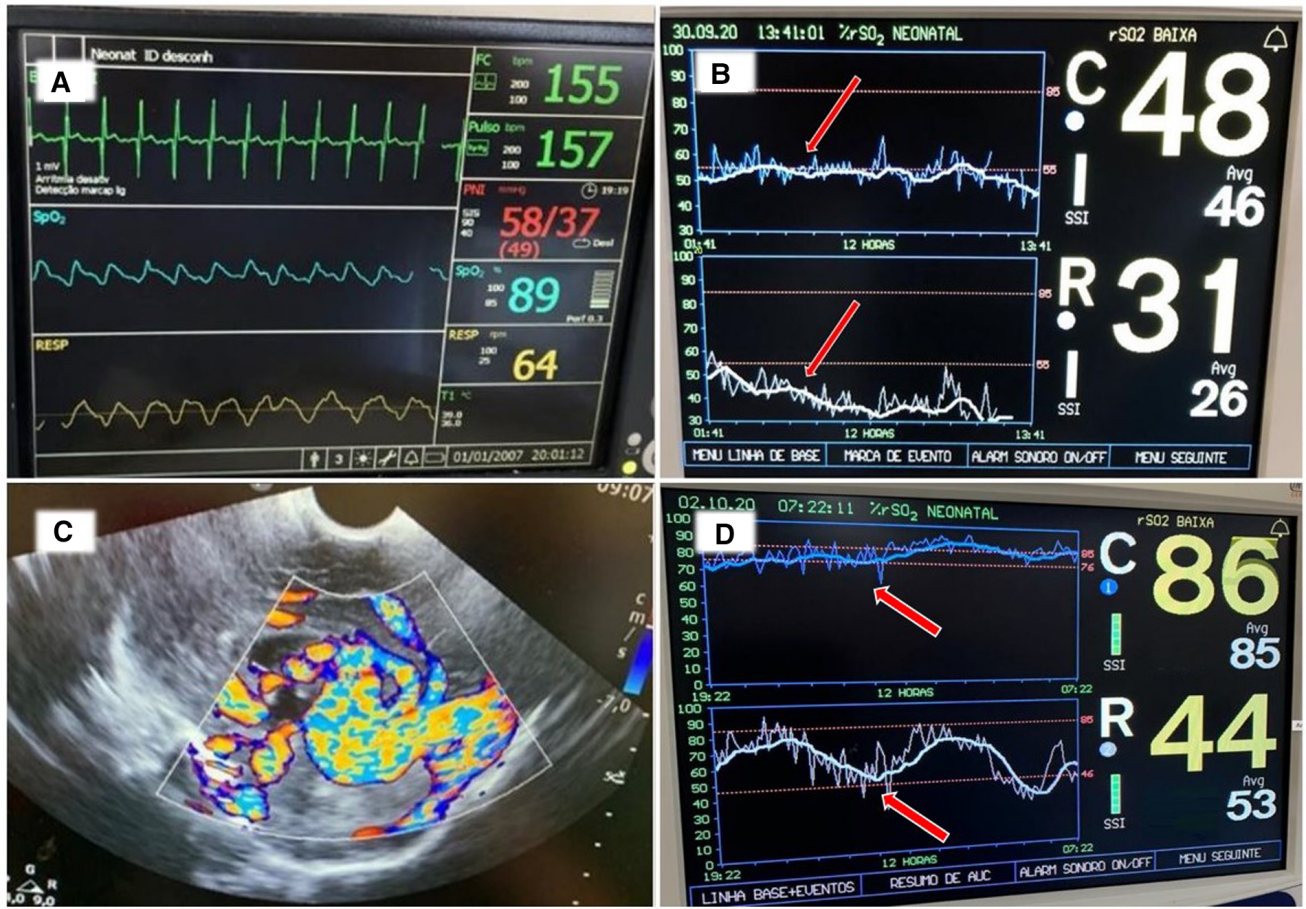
(A) Vital signs monitor with values within the normal range. (B) NIRS monitoring on the second day of life shows rScO_2_ (blue trend line) ranging 46%–50% while rSrO_2_ (white trend line) progressively declined with marked variability seen in both sites (arrows). (C) Cranial ultrasound showed an anechoic area located in the midline and associated with high flow compatible with vein of Galen aneurysmal malformation. (D) NIRS monitoring on day of life 5 shows an increase in both rScO_2_ (blue line) and also in rSrO_2_ (white line), but with marked variability in rSrO_2_. The infant received a PRBC transfusion due to a Hct 27% (arrows).
